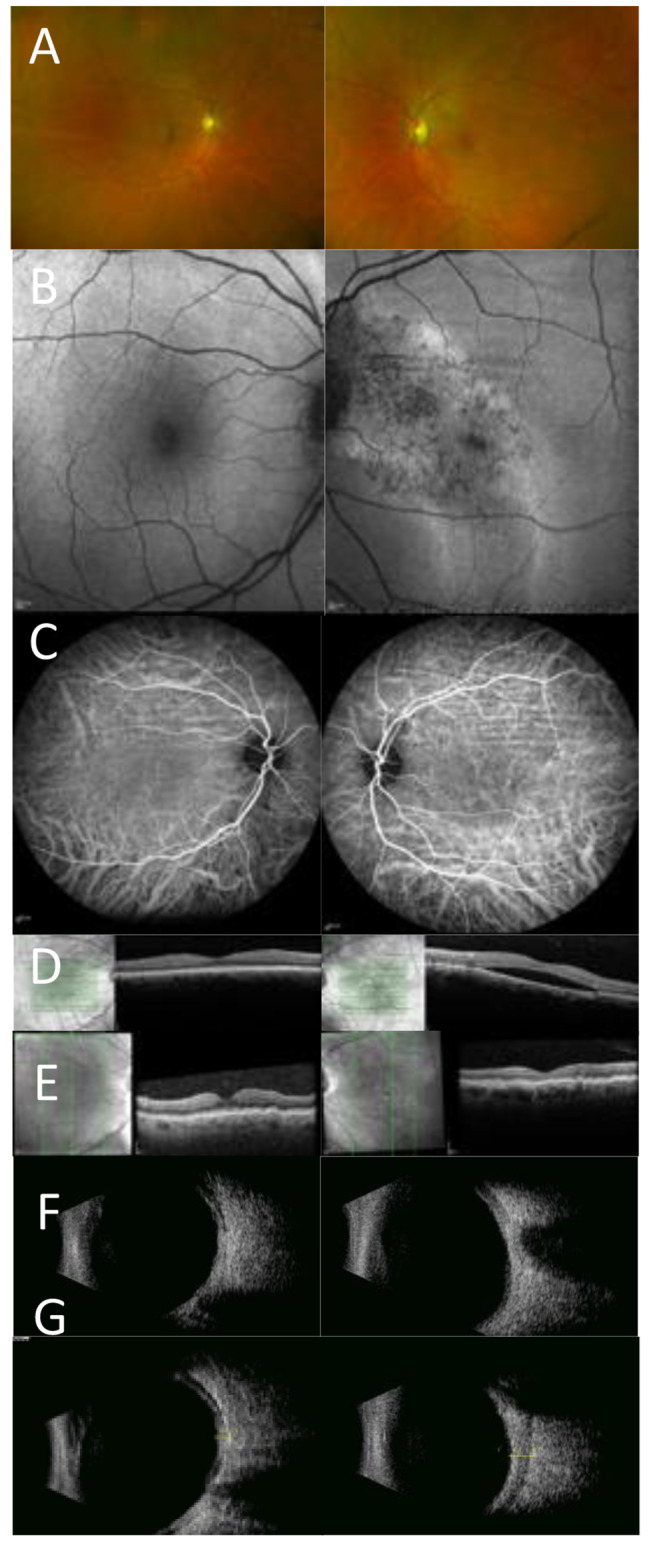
Multi-modal imaging for case 2. A) Optos imaging showing CRF in the macular region; more pronounced in the left eye B) FAF imaging showing features of chronic CSC with gravitational tract in the left eye, and CRF that are more pronounced in the left eye (C) ICGA imaging showing bilateral CRF (D-E) OCT imaging using horizontal scan at baseline(D) showing serous fluid in the central macula of the left eye and follow up OCT vertical scan (E) showing bilateral chorioretinal folds with resolution of the CSC neurosensory retinal elevation (F) Ultrasound B scan showing bilateral flattened posterior poles. (G) and thickened ocular coats in the left eye
